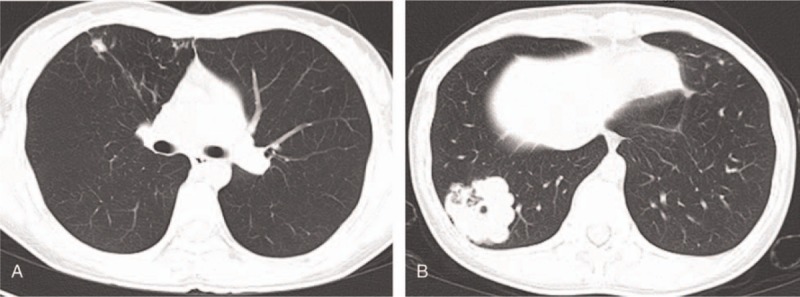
Computed tomography of the chest revealing (A) irregular nodular lesions in the right upper lobe and (B) a well-demarcated, solitary lung mass located in the right lower lobe peripherally.
